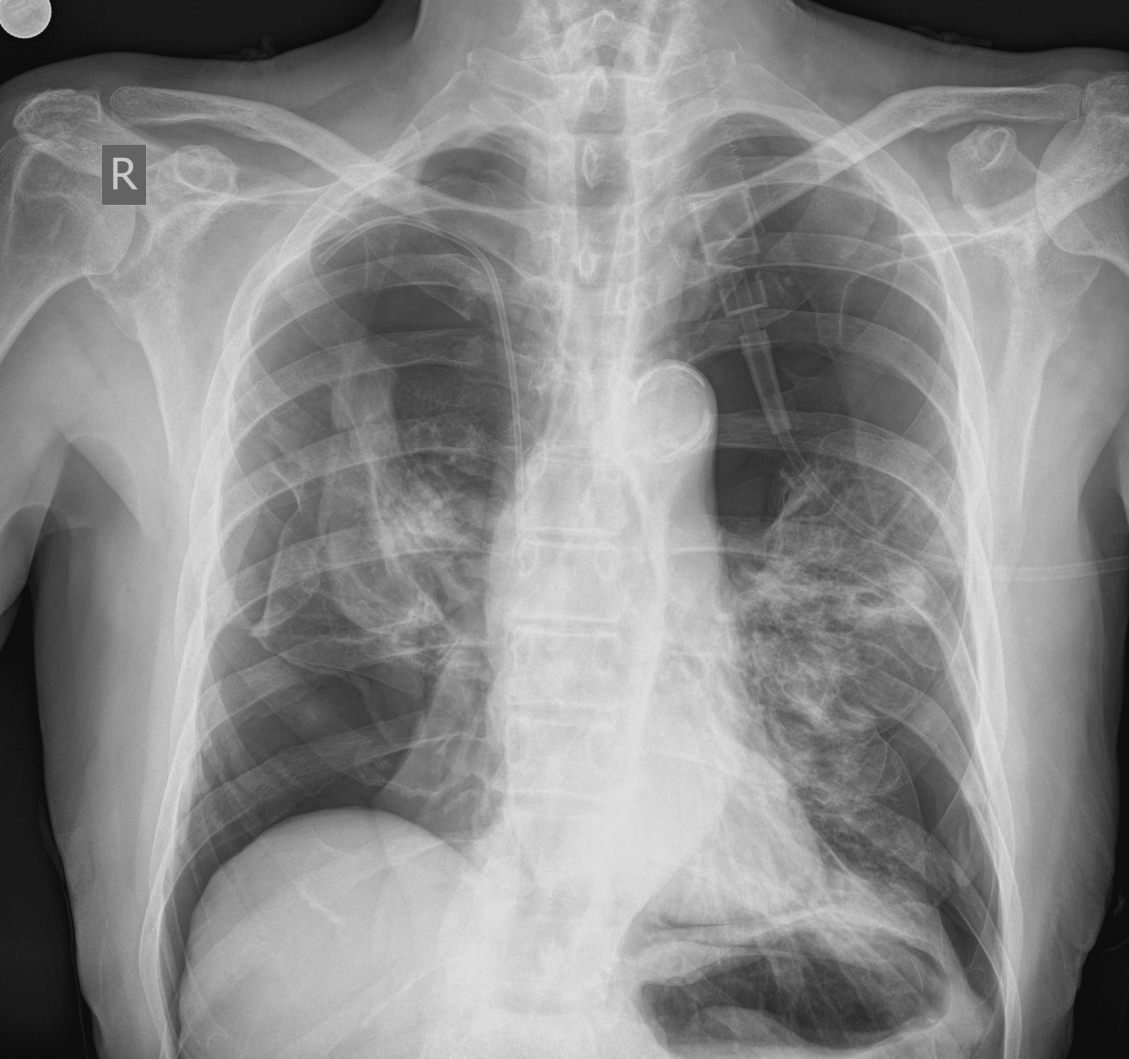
Chest X-ray of a 74-year-old male patient with leiomyosarcoma and lung metastases treated with pazopanib, demonstrating bilateral tension pneumothorax upon presentation to the emergency department.
